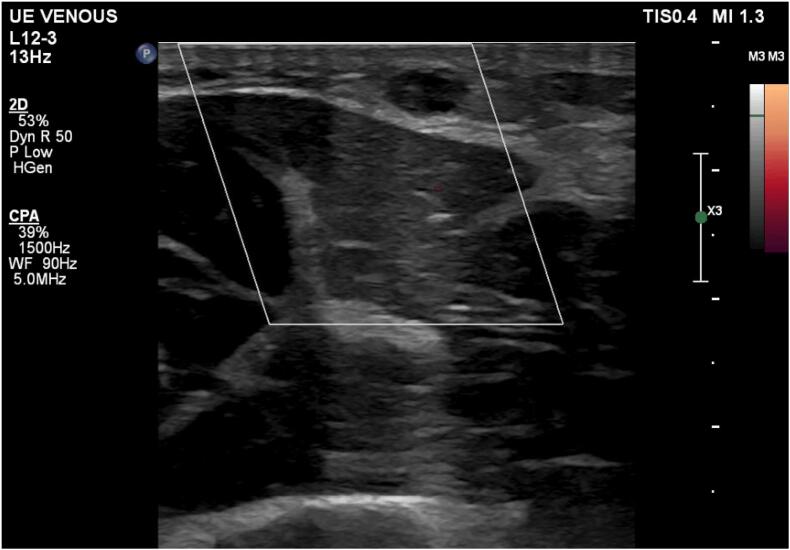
Duplex ultrasound image of the left arm showing a superficial vein thrombosis in the cephalic vein.
